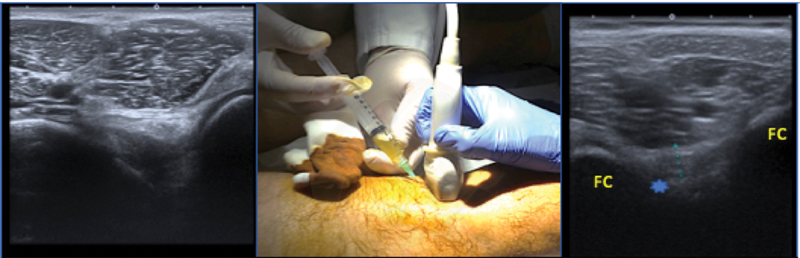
Ultrasound-guided injection of PRGF Endoret (BTI) inside the posterior cruciate ligament sheath (Courtesy of Dr. Marta Rius and Dr. Ramón Cugat). *Determines axial view of posterior cruciate ligament body. FC, femoral condyle.
